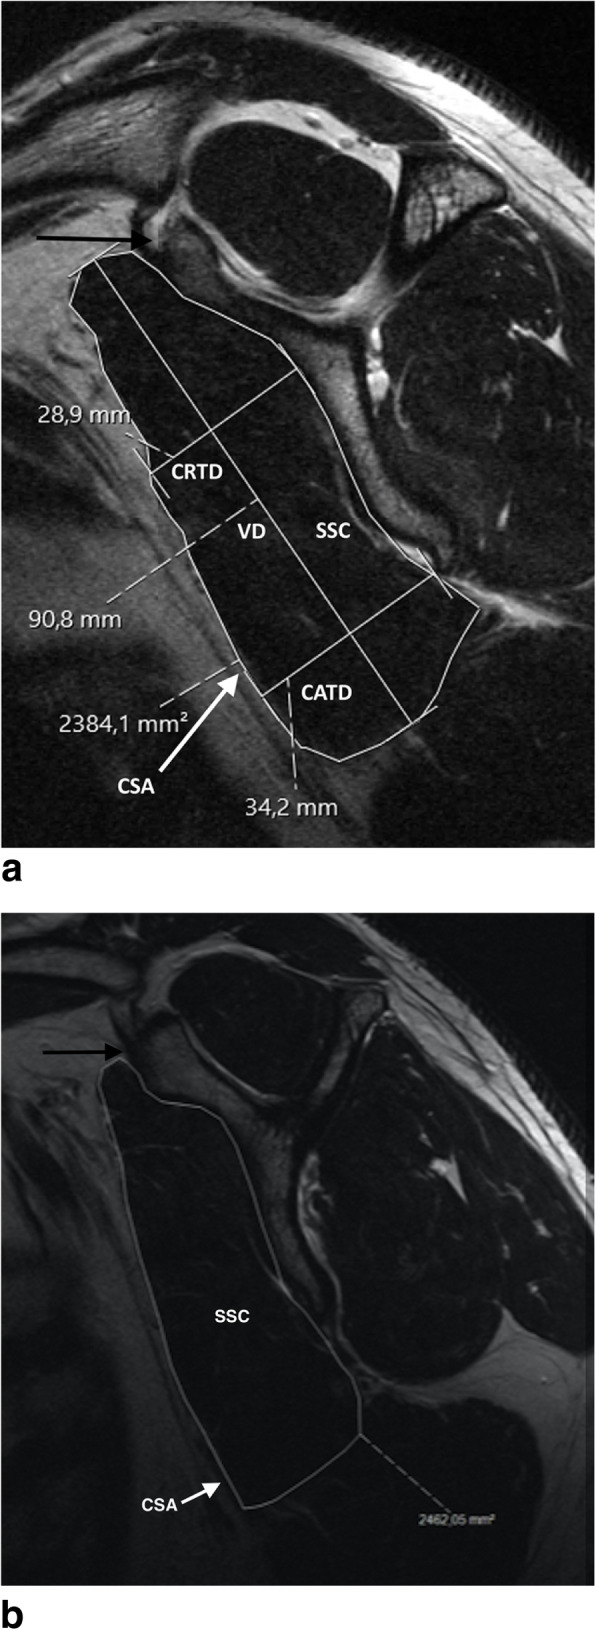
a Healthy subscapularis muscle - measuring of the vertical diameter (VD), cranial transverse diameter (CRTD), caudal transverse diameter (CATD) and cross sectional area (CSA); SSC: subscapularis muscle; black arrow: ventral tip of the scapular “Y”. b “Y-position” of the MRI sagittal oblique plane: Healthy subscapularis muscle - measuring of the cross sectional area (CSA); SSC: subscapularis muscle; arrow/CSA: cross sectional area; black arrow: ventral tip of the scapular “Y”
